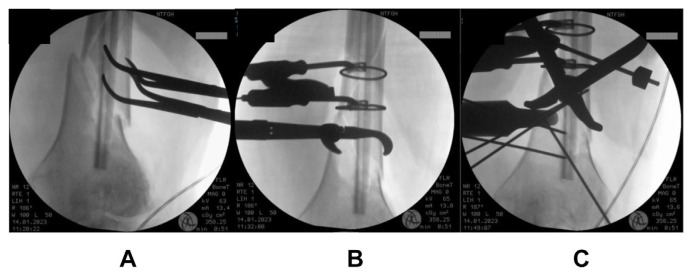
Intraoperative radiographs show the fibular strut allograft placed within the fracture site, before reduction and temporary fixation with K wires.
